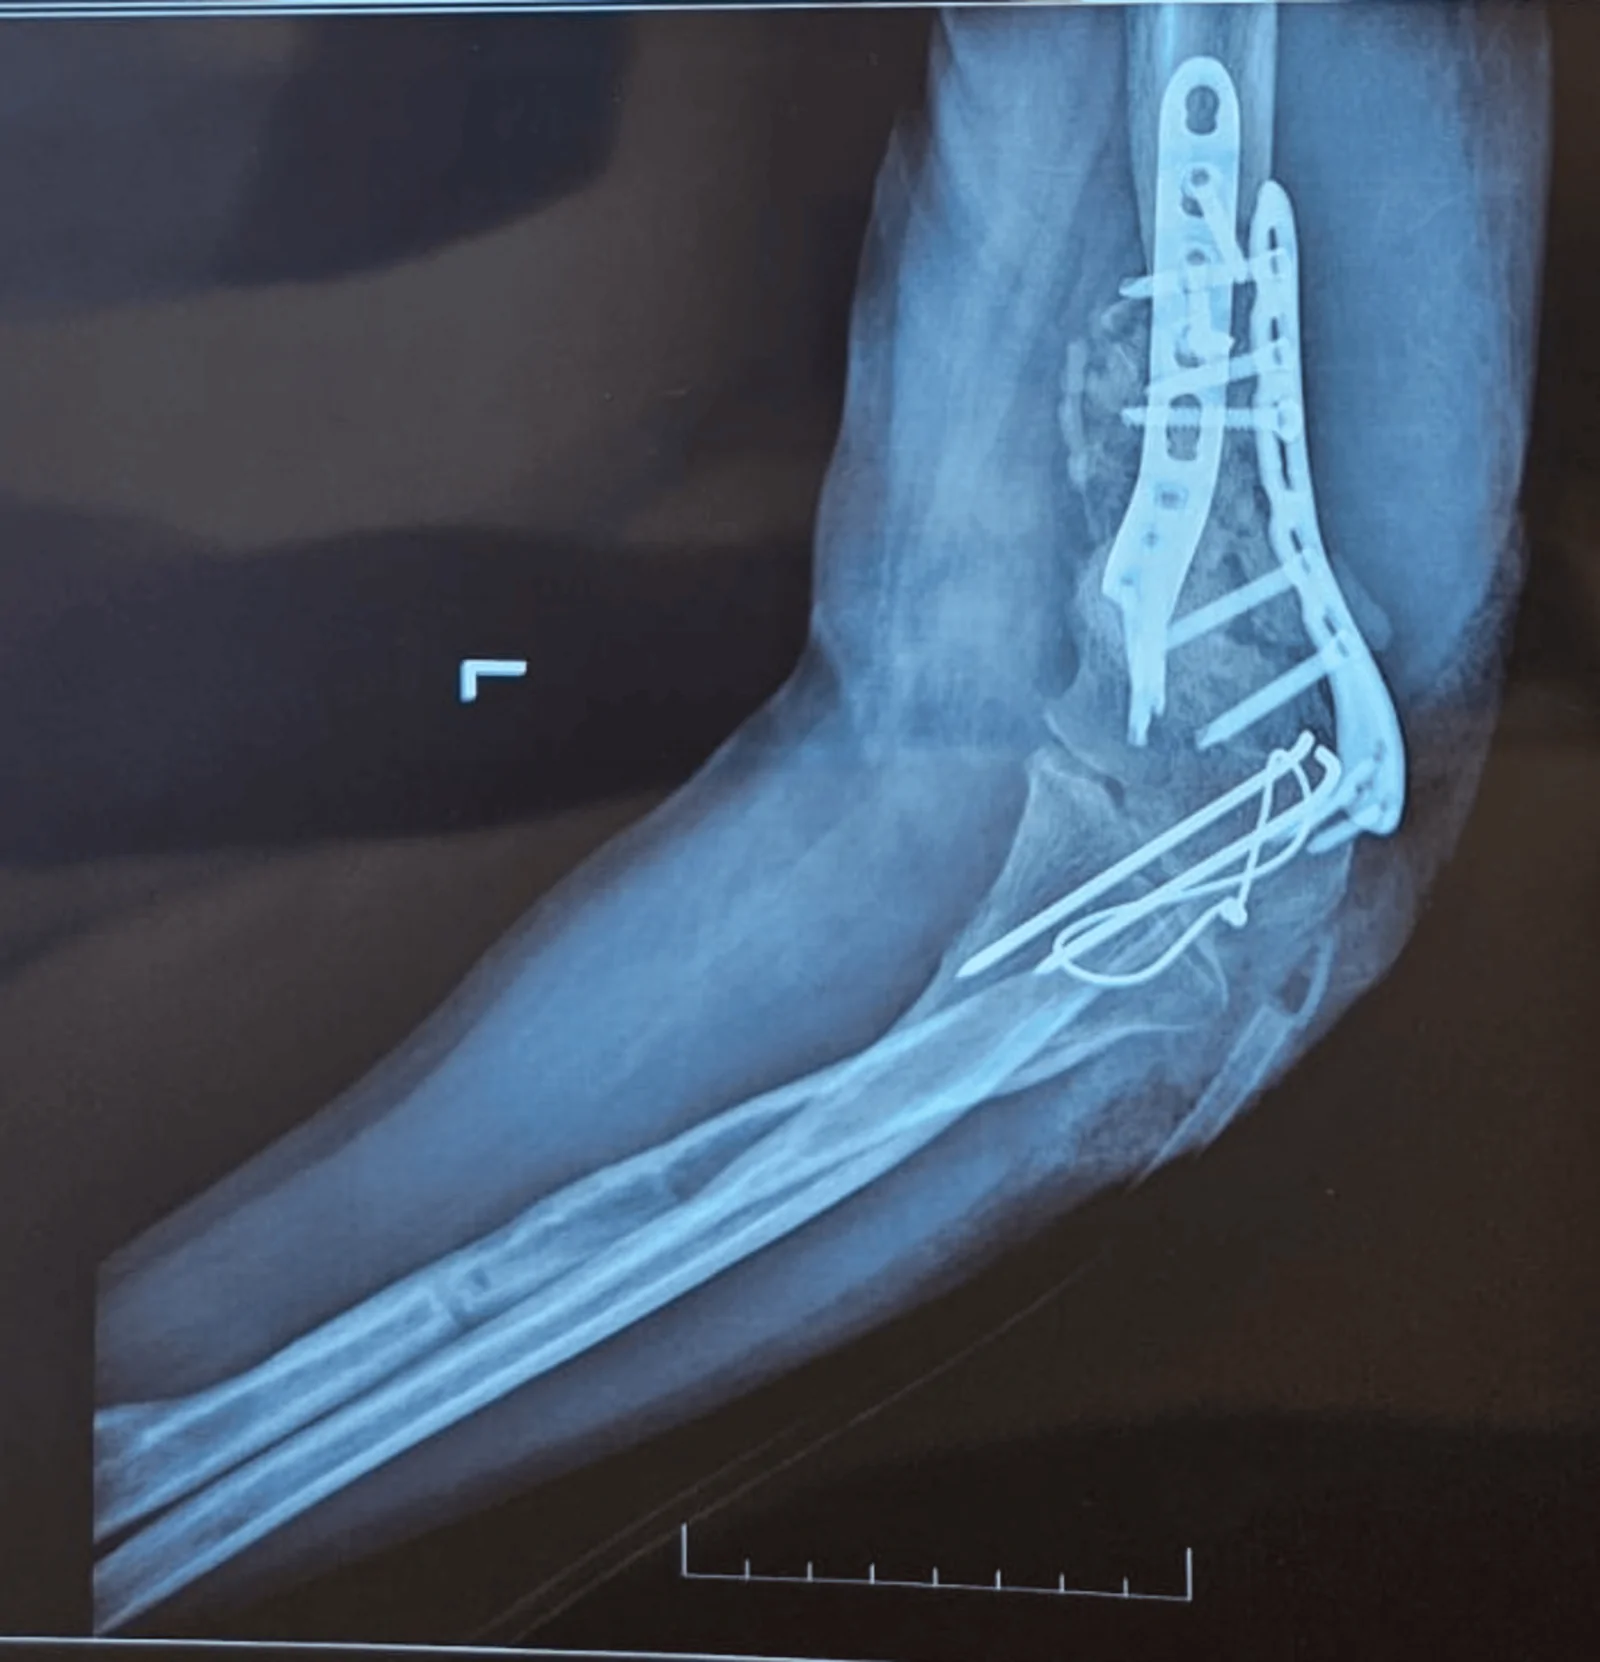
Plain radiographs of the elbow showing retained distal humerus implants with features suggestive of chronic implant-associated infection
